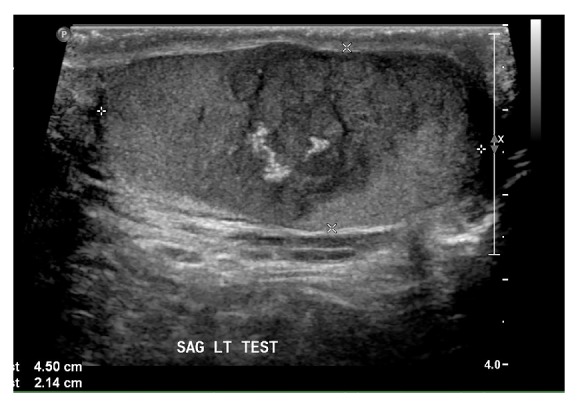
Sagittal sonogram of the left testis demonstrates testicular mass with calcifications.
